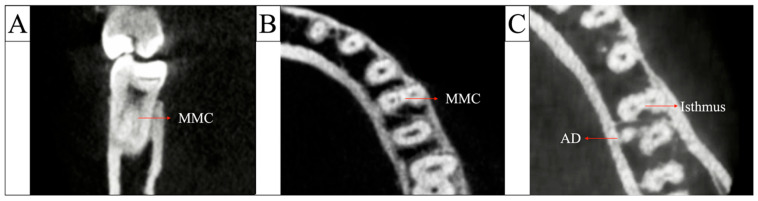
(A) Coronal CBCT slice showing a distinct middle mesial canal (MMC) between the MB and ML canals. (B) Axial view confirming the MMC as a separate canal within the mesial root. (C) Axial section demonstrating a mesial root isthmus and the presence of an accessory distal root (AD) compared with the main distal root.
